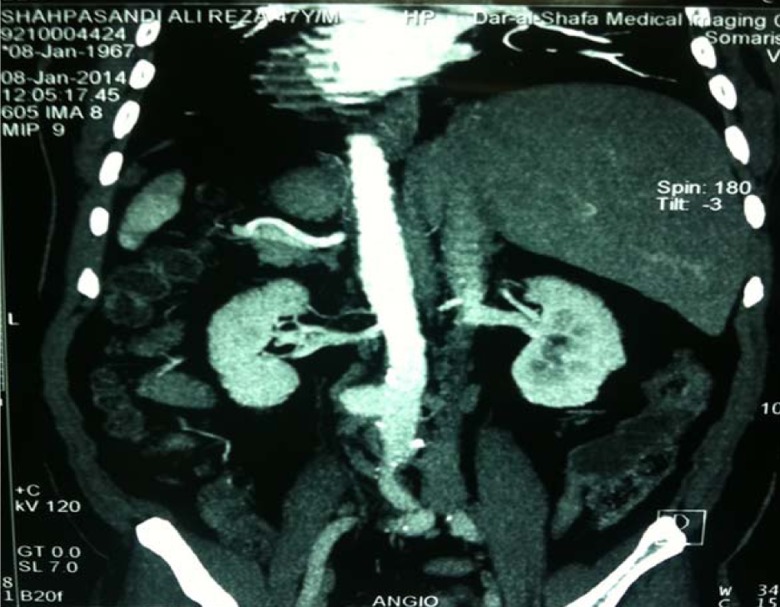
CT-angio revealed two aneurysms on descending thoracic aorta, abdominal aorta and Aortoentric fistula between abdominal aneurysm and duodenum.
